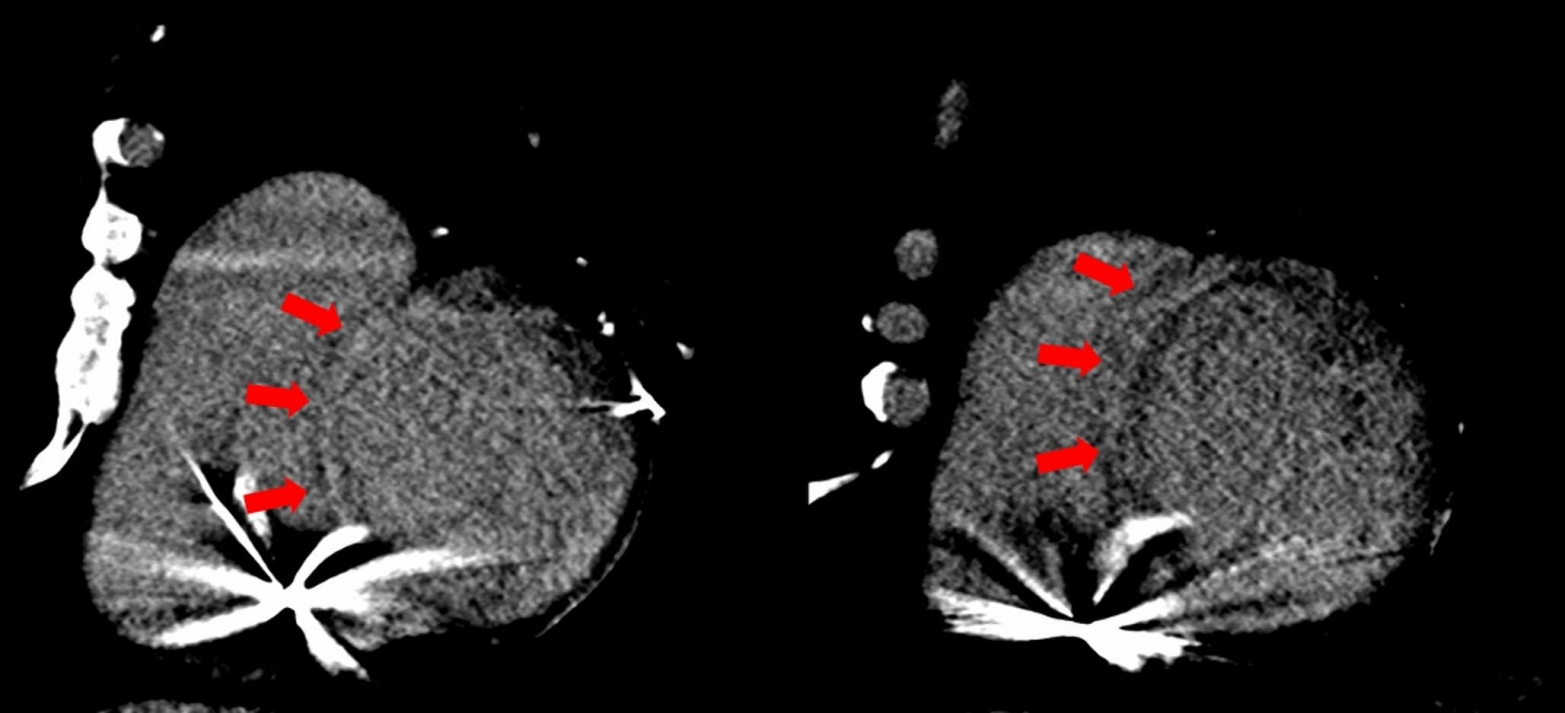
Tissue Characterization with late iodine enhancement (LIE) imaging in a Patient with Ischemic Dilated Cardiomyopathy and a CMR-Incompatible Device. Short-axis cardiac CT images acquired during the delayed phase demonstrate LIE with a transmural distribution, consistent with ischemic scar involving the septal walls
